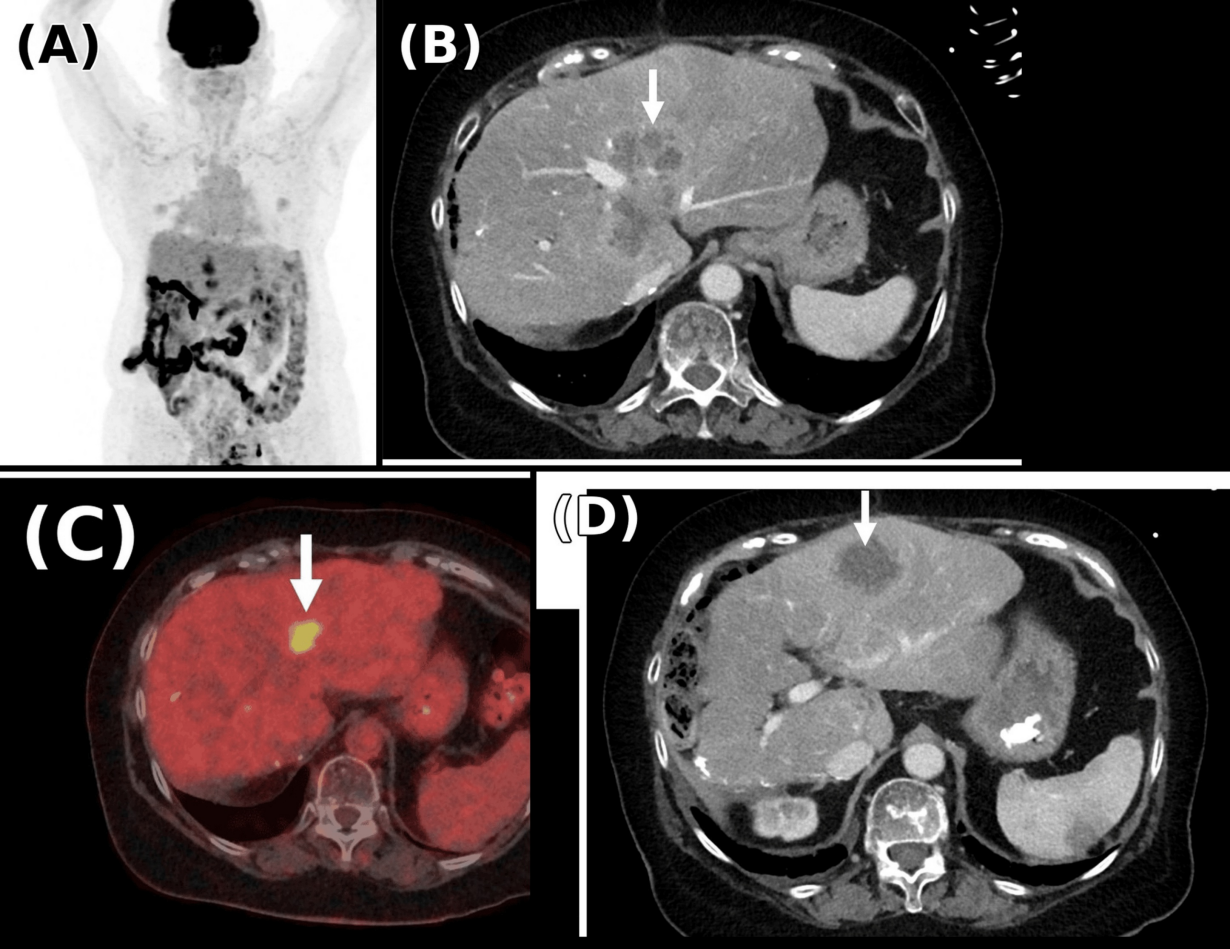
FDG-PET/CT imaging from February 2022 (A) Coronal whole-body FDG-PET showing widespread metabolic activity. (B) Axial CT showing hepatic metastatic deposits with post-ablation changes (arrow). (C) Axial FDG-PET/CT fusion showing a focal hypermetabolic hepatic lesion (arrow). (D) Axial CT at a lower level showing additional hepatic metastatic deposits (arrow). FDG-PET/CT: fluorodeoxyglucose-positron emission tomography/computed tomography
